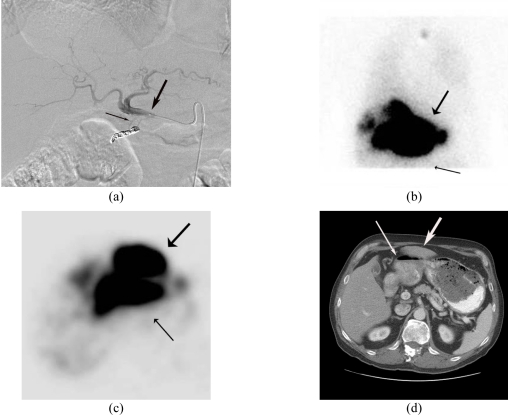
67 year old male with hepatic metastatic colorectal cancer undergoing pre-treatment hepatopulmonary shunt evaluation. (a) Proper Hepatic Arteriogram; Tc99 MAA was injected via a microcatheter placed in the proper hepatic artery (arrow) following coil embolisation of the gastroduodenal artery; Note opacification of the patent right gastric artery (arrow heads). (b) Planar thoracoabdominal scintigraphy following hepatic arterial injection of Tc99 MAA demonstrates predominant left lobe hepatic activity only (bold arrow) and absence of extrahepatic activity (thin arrow). (c) SPECT images (a) demonstrate activity corresponding to the CT (b) images of the left lobe of the liver (bold arrow) and in the stomach (thin arrow) not noted on the planar image.
